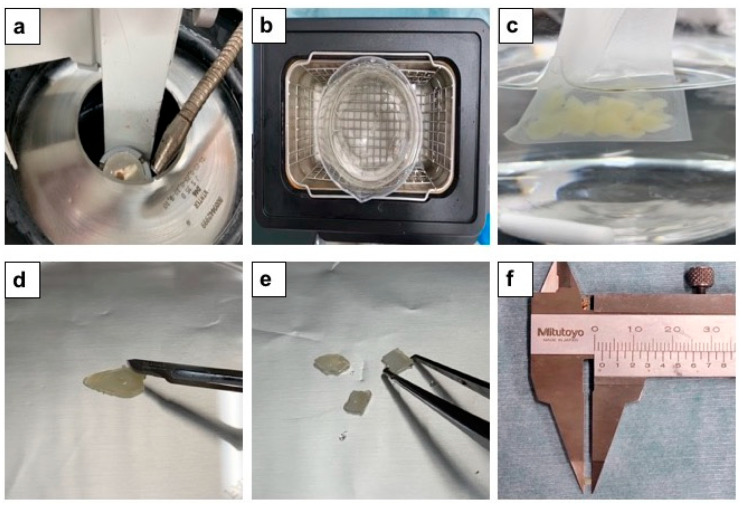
Preparation of human dentin blocks. (a) Dentin-root portion was sliced into several disks (1.0 mm in thickness) by using electrically rotating cutting machine (Leica SP1600 Germany), (b) Ultrasonic demineralization in 2% HNO_3_ liquid (1.0 L) for 30 min, (c) Ultrasonic washing in D.W. (1.0 L) for 30 min, (d–f) Demineralized dentin disks were cut into blocks (2 × 2 × 1 mm^3^).
